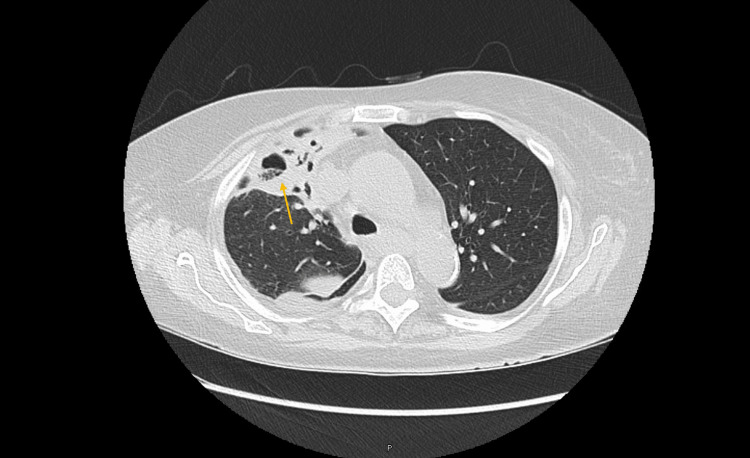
CT chest on admission The yellow arrow shows large right upper lobe consolidation.
